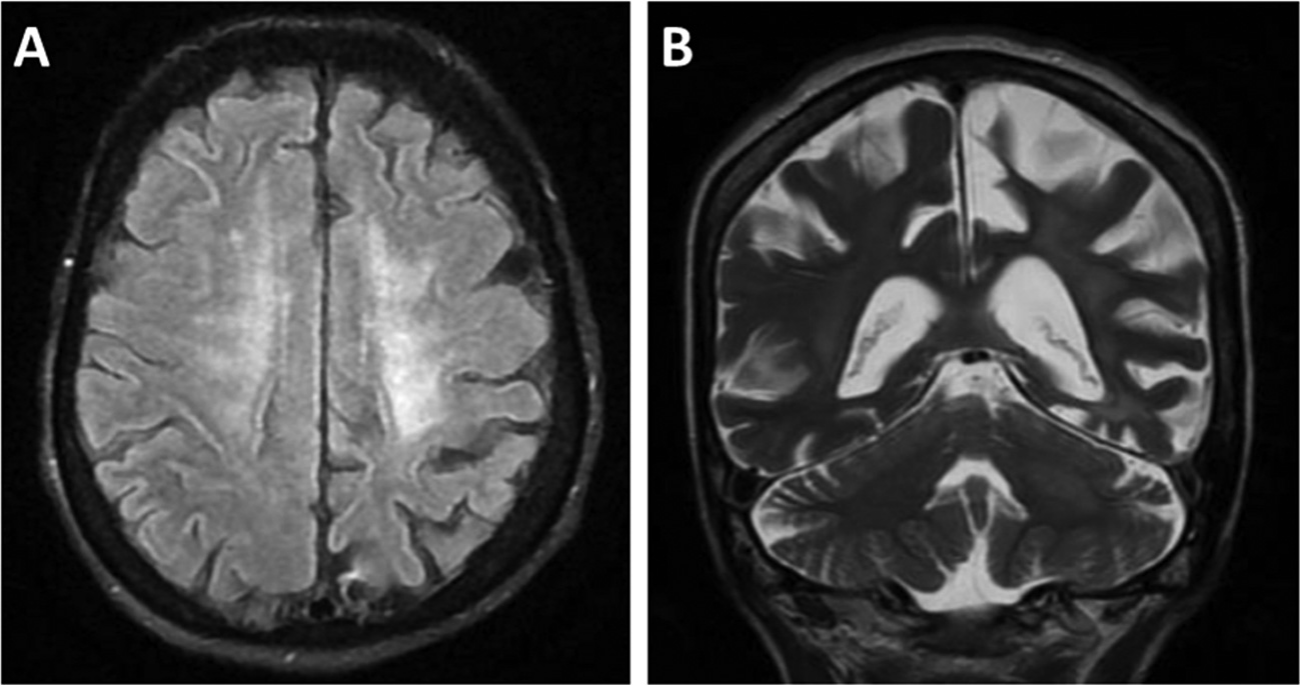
(A) Magnetic resonance imaging with axial T2/FLAIR sequence shows nonspecific hyperintensities in the white matter. (B) Coronal T2-weighted sequence shows brain volumetric reduction predominantly on the left parieto-occipital region, hyperintensities in the white matter, and moderate dilatation of supratentorial ventricular system with prominence of the cerebral sulci and basal cisterns.
